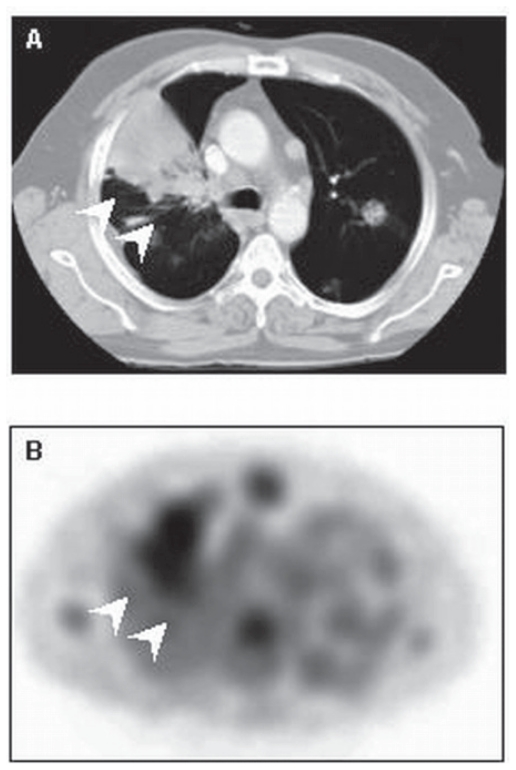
A 52-year old man with NSCLC and post-obstructive pneumonia in the right upper lobe (white arrows) on contrast-enhanced CT (A) which shows intense tracer uptake (white arrows) in the baseline Annexin V SPECT (B).
